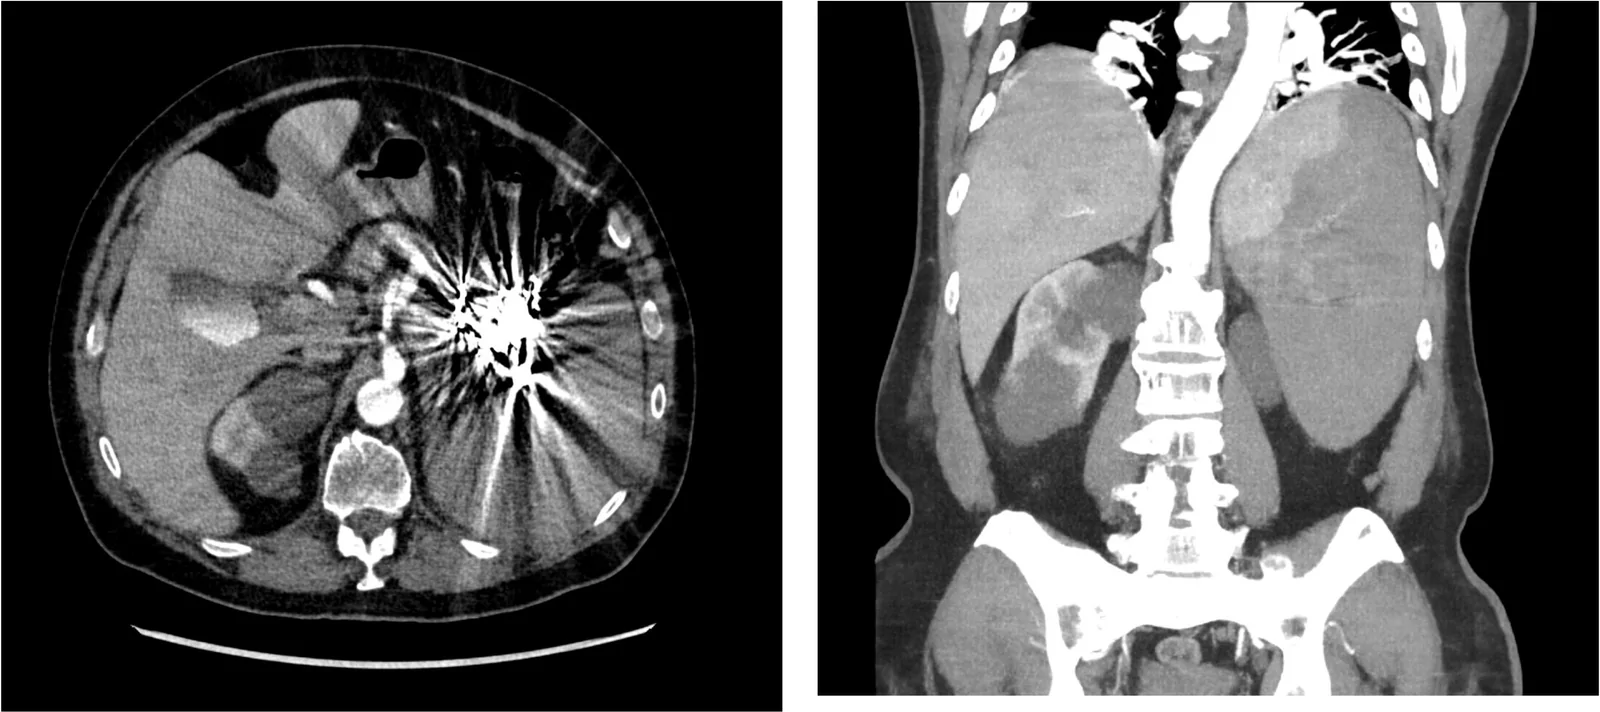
Follow-up CT angiogram axial and coronal views 1 day post procedure demonstrate expected evolving post embolization changes to the splenic parenchyma including a large hypodense nonenhancing region within the majority of the spleen.
